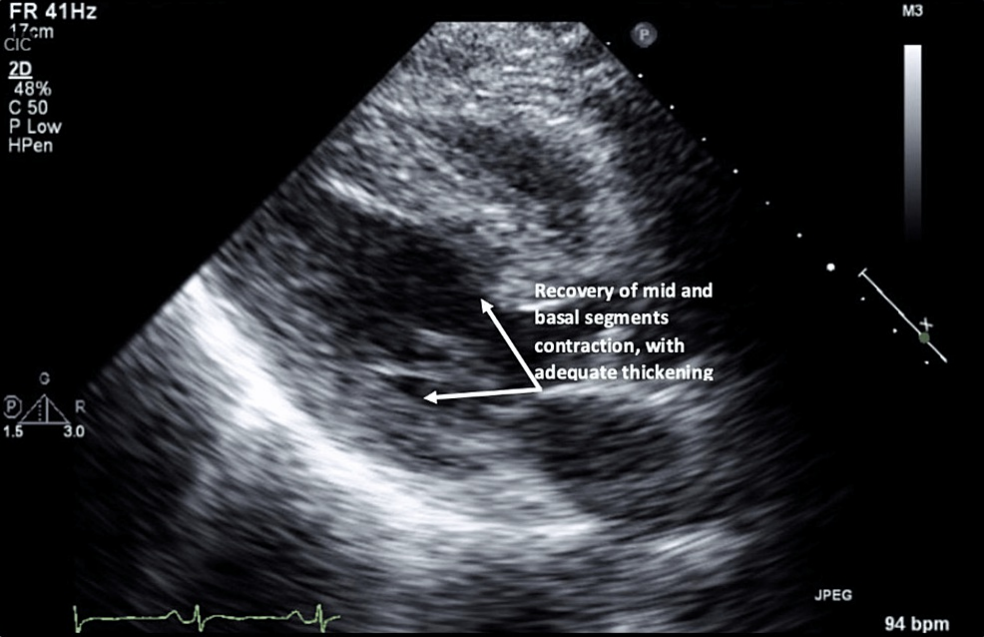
Transthoracic echocardiogram, parasternal long axis view at the end of the systole showing fully contracting apex along with recovery of the basal and mid segments contractile function.
